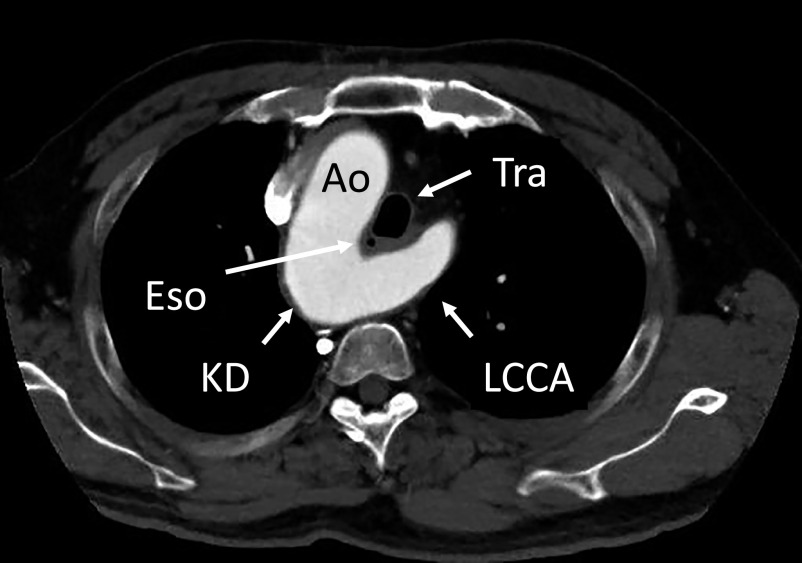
Axial computed tomography angiography showing a 26-mm aneurysmal Kommerell diverticulum (KD) with right aortic arch (Ao). The esophagus (Eso) is compressed by the trachea (Tra), and the KD connects to the common carotid artery (LCCA).
